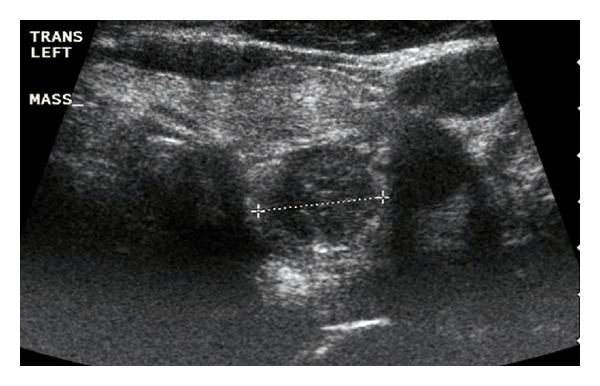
Ultrasound number 1 of left lower parathyroid gland—7/24/12: after infarction.
